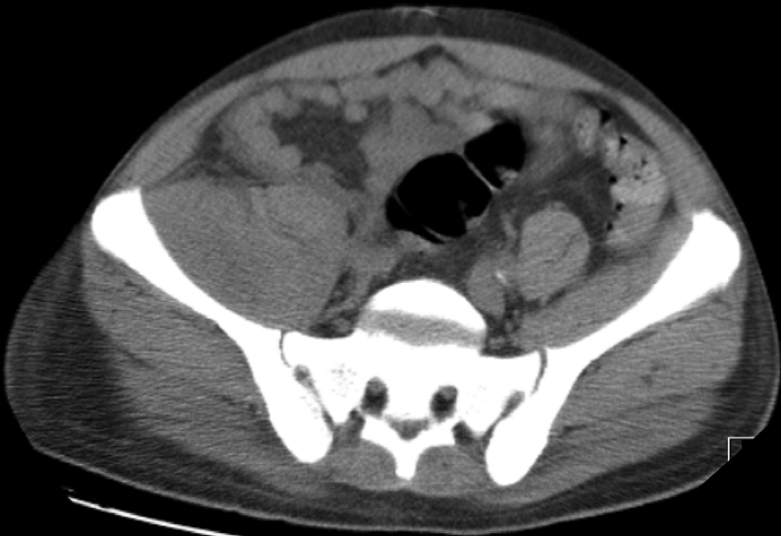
A CT scan showing hypertrophy of the psoas muscle on the right side.
